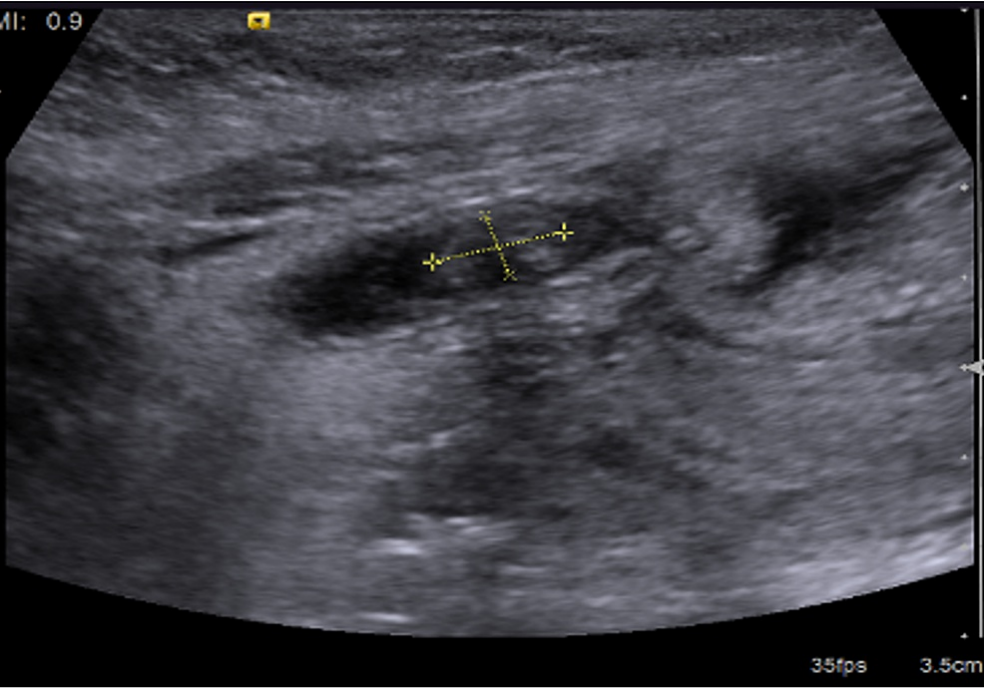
Abdominal ultrasound was consistent with inflamed left-sided appendix
